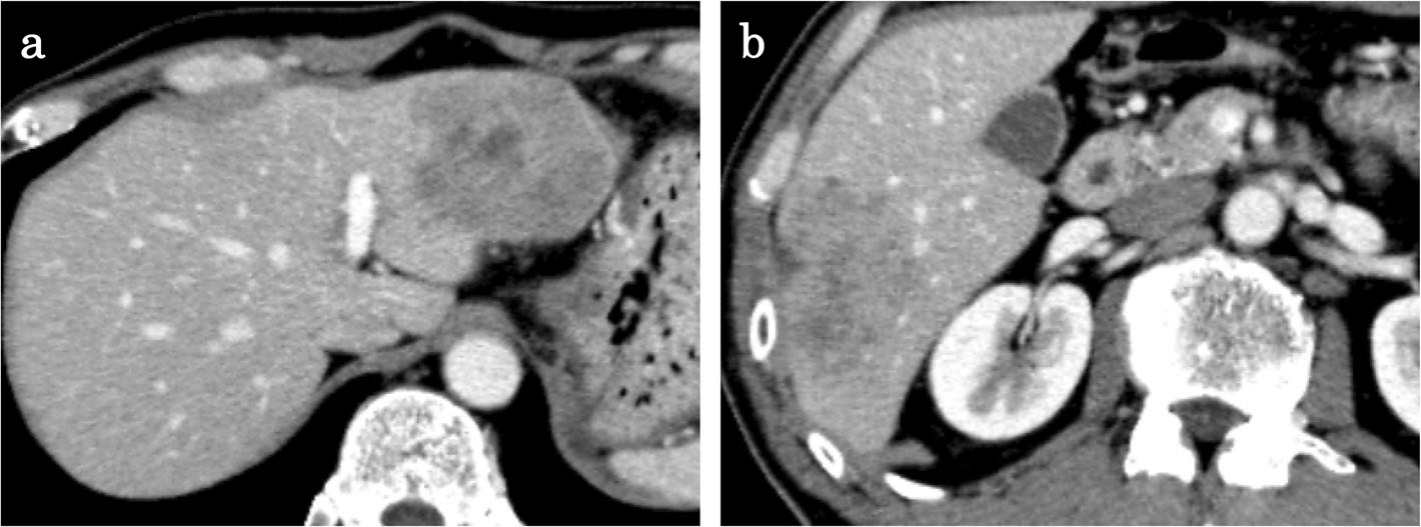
Liver nodules before chemotherapy. a, b Enhanced CT scan showed irregular low-density nodules in segment 2/3 (a, 60 mm in diameter) and segment 6 (b, 57 mm in diameter), which were suspected to be liver metastases
